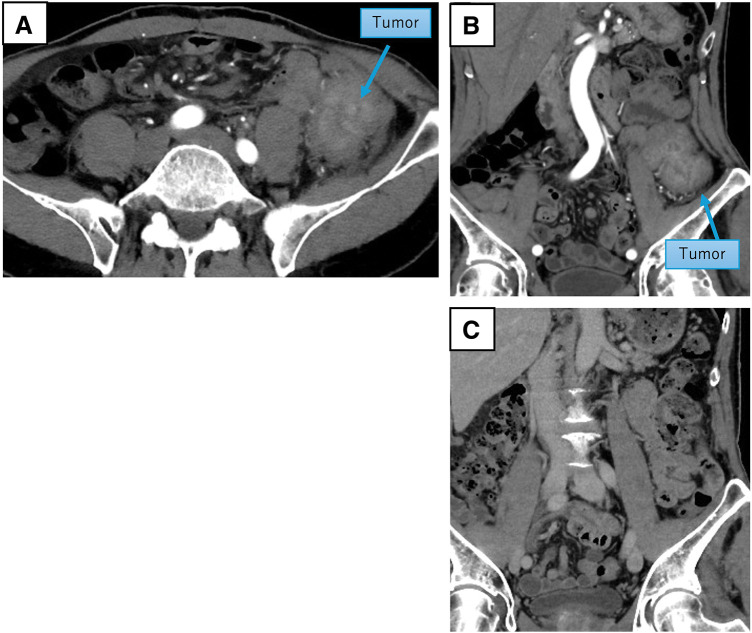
Preoperative abdominal contrast CT. Preoperative images show a 50 × 50 × 60-mm mass (blue arrows) with wall thickening in the mid-descending colon, raising the suspicion of infiltration into the adjacent small intestine and abdominal wall (A, B). A CT image (C) obtained 1 year prior to prostate surgery, demonstrating no masses in the descending colon.
